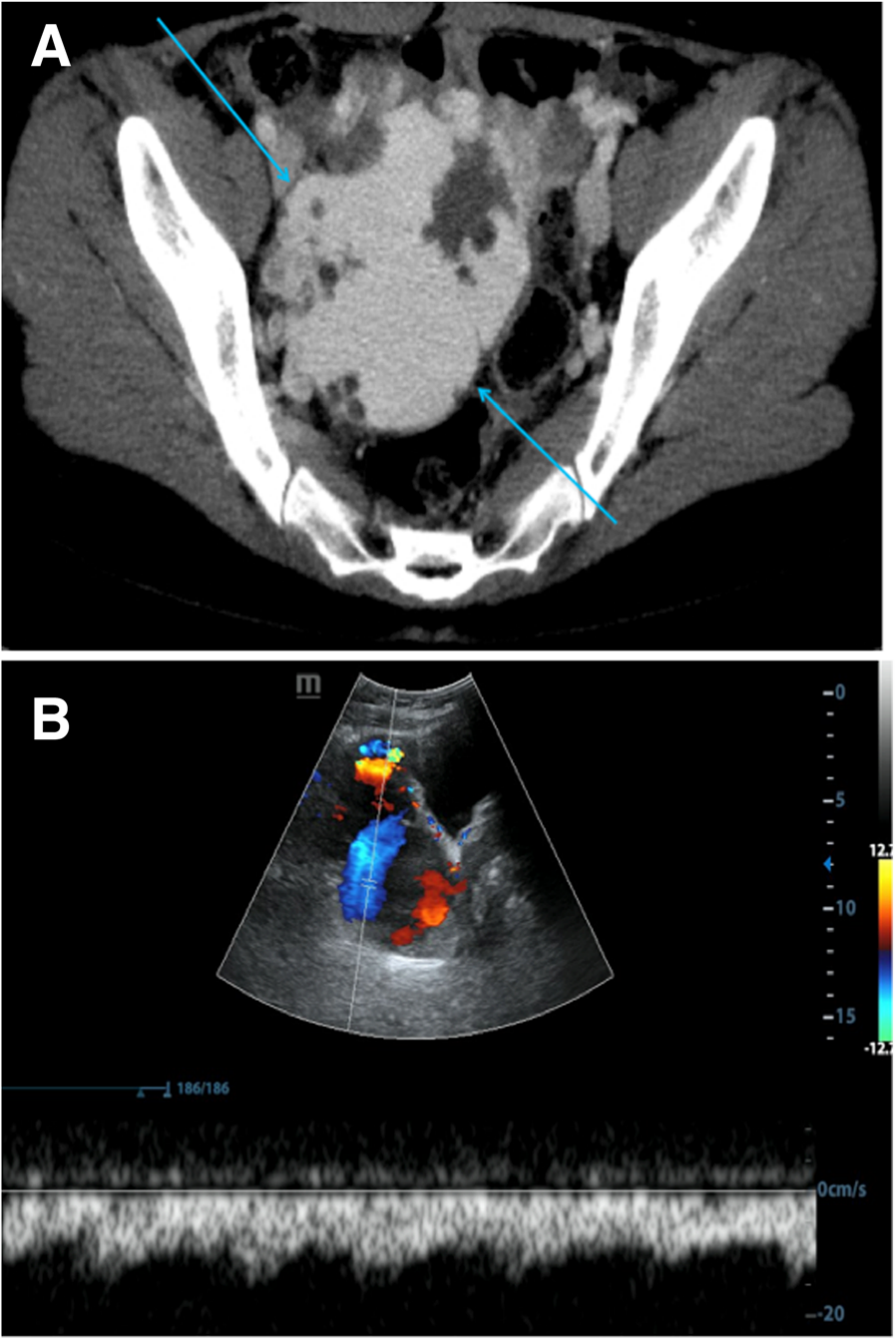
Contrast-enhanced MDCT shows (a) a suspicious giant pelvic AVF (arrows), (b) confirmed by Color Doppler
